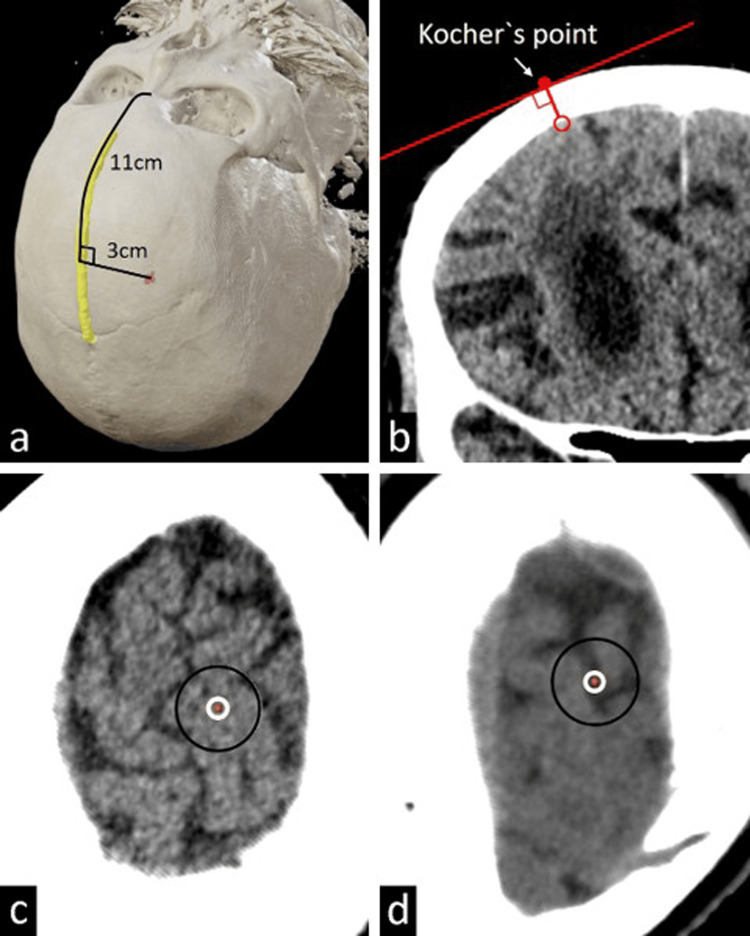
Computed tomography images with Brainlab Elements software. Kocher’s point was determined as 11 cm posteriorly from the nasion along the skull surface and 3 cm laterally from the midline (a). The reference entry point was detected as the intersection of a perpendicular line from Kocher’s point and the cerebral surface (b). A case of a sufficient margin to reference the entry point from the cerebral sulci (c). A case that required adjustment of the entry point for ideal ventriculostomy (d). White circle: 2.5-mm radius from the reference entry point. Black circle: 7.5-mm radius from the reference entry point (burr hole edge).
